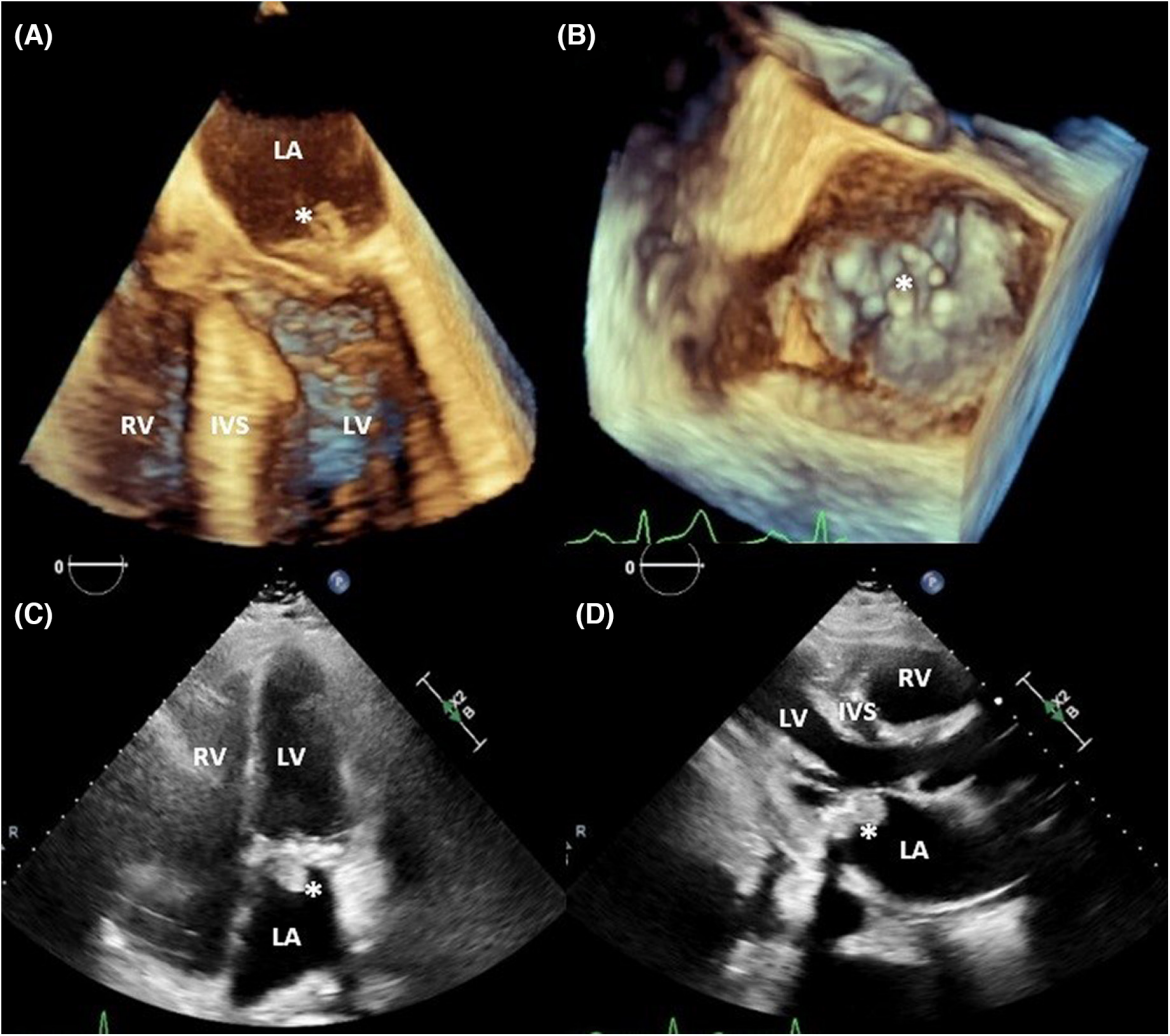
Three‐dimensional transesophageal echocardiography showing mobile serpiginous vegetations on the atrial aspect of both anterior and posterior mitral leaflets (A, B). Follow‐up transthoracic echocardiography showing calcified vegetation measuring 2 × 1.5 cm at the tip of posterior mitral leaflet (C, D). IVS, interventricular septum; LA, left atrium; LV, left ventricle; RV, right ventricle; *vegetation.
